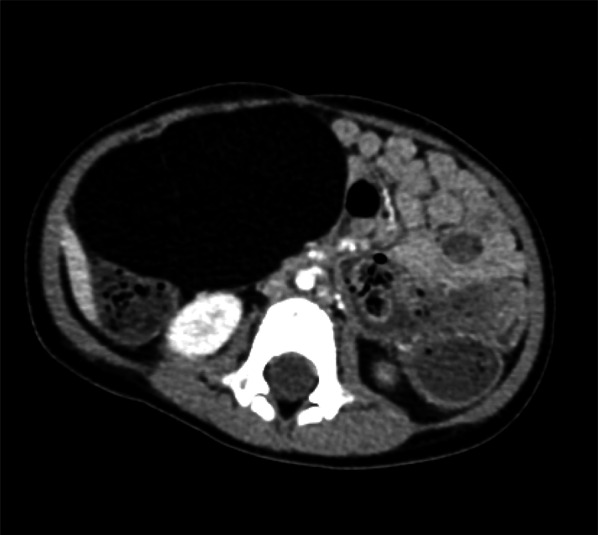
Computed tomography obtained a large low-density mass within the mesentery, measuring approximately 8 × 6 cm
